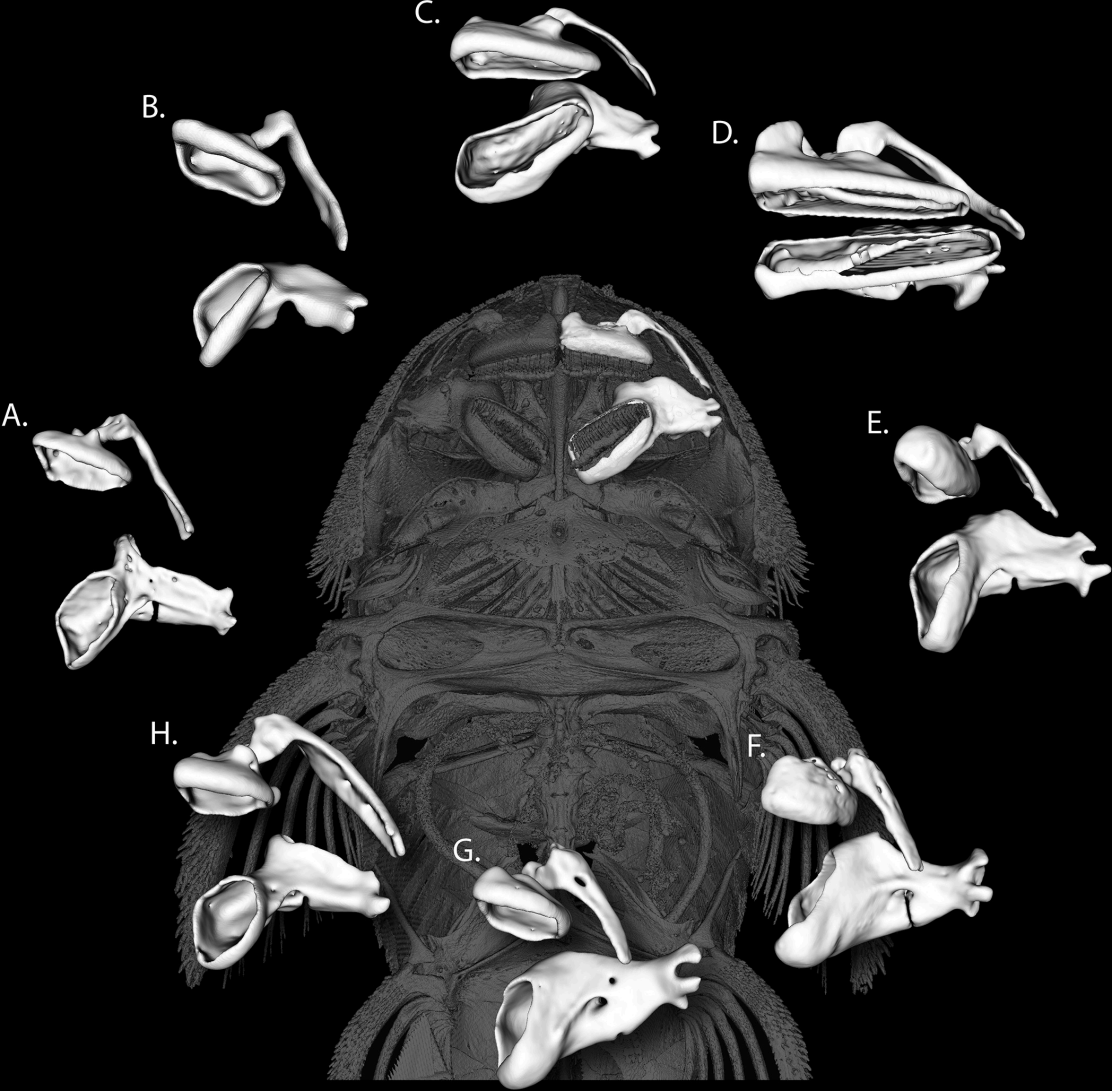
Oral jaw shape diversity in loricariid catfishes. The left oral jaws are shown in white. In the center, the placement of the oral jaws are shown for *Baryancistrus xanthellus*, MorphoSource ID: M37989-69325, Collection ID: ANSP 198216. Representatives of other species are as listed; A) *Otocinclus vittatus*, M39000-70695, ANSP 174732, B) *Farlowella acus*, M37599-68848, ANSP 191381, C) *Harttia loricariformis*, M44340-80321, ANSP 190961, D) *Chaetostoma milesi*, M24790-48802, AUM Unknown ID, E) *Panaqolus*, M37593-68839, ANSP 194642, F) *Hypancistrus vandragti*, M37980-69316, ANSP 199002, G) *Hypancistrus zebra*, M37572-68813, ANSP 197839, and H) *Microlepidogaster perforates*, M37975-69310, ANSP 174117. All CT scans were obtained from MorphoSource.
